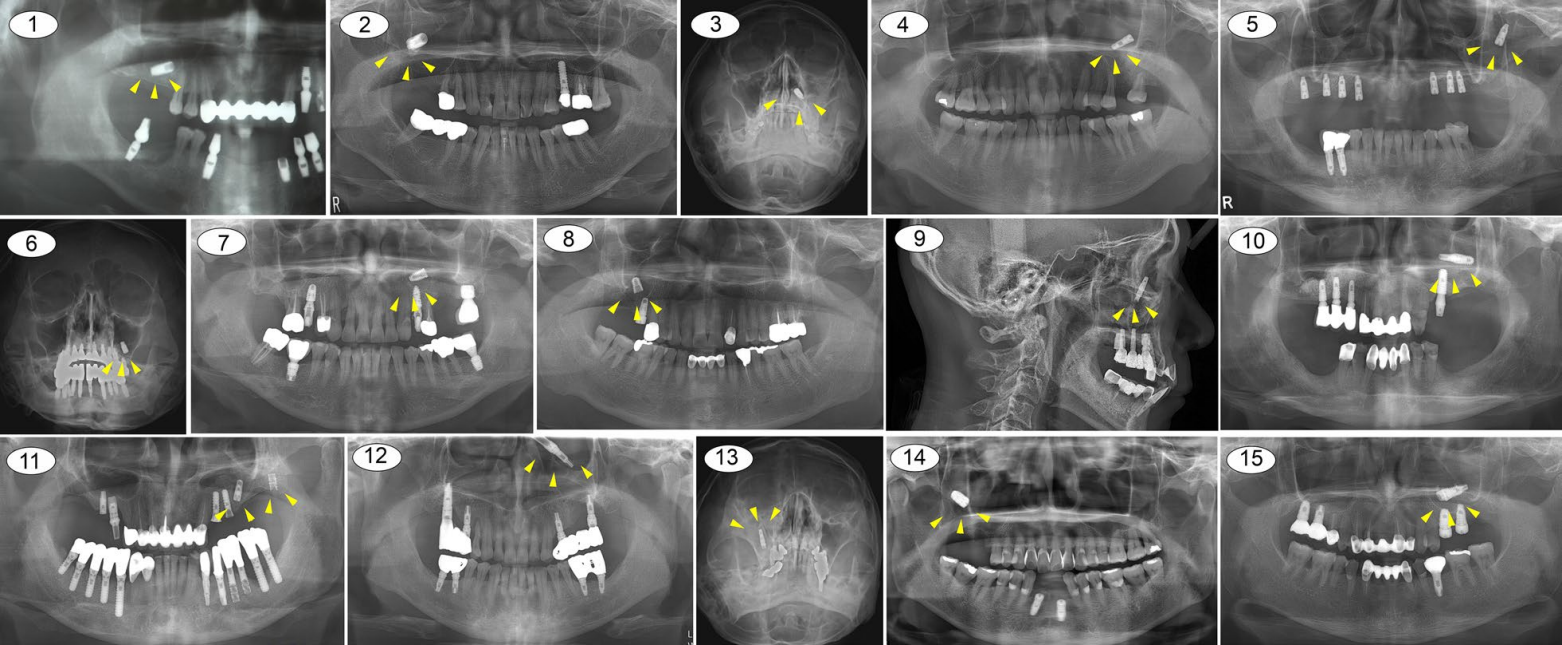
The preoperative radiographic evaluation visualizing displaced dental implants on panoramic radiographs, Water’s view, posteroanterior and lateral cephalograms, and computed tomography of the 15 patients included in this study
